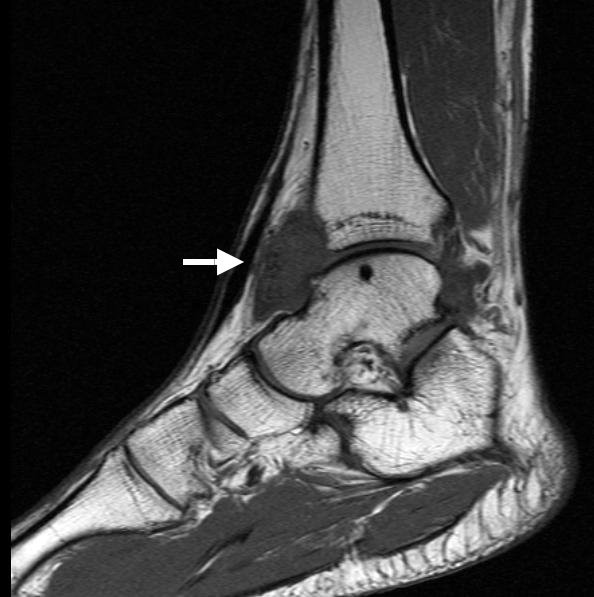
Sagittal T1-weighted spin-echo MRI of the right ankle showing a well delineated giant cell tumor anterior to the ankle (arrow).
